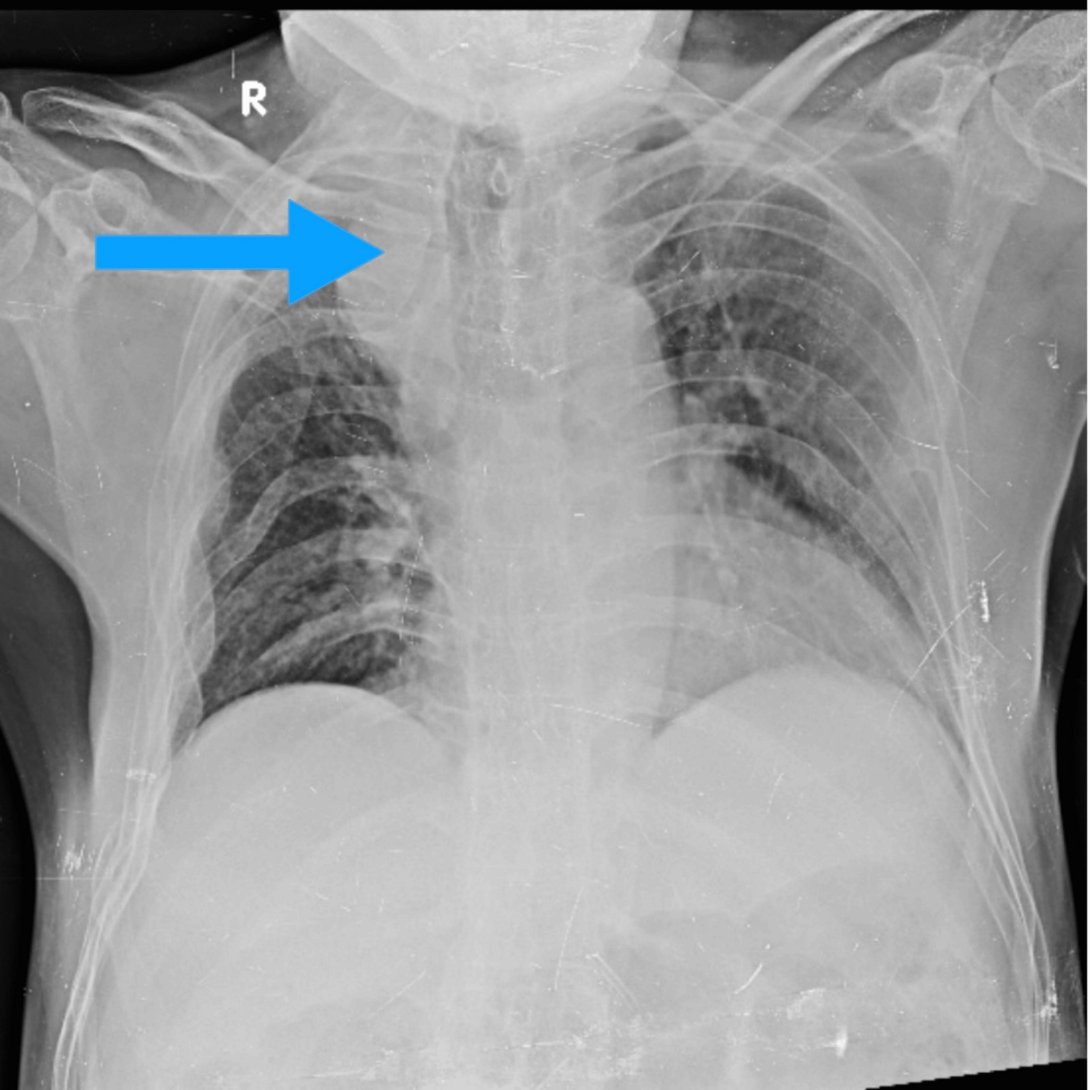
AP chest radiograph showing increased upper thoracic kyphosis. There is a probable right paratracheal opacity mass with tracheal deviation The blue arrow shows the opacity which might indicate the mass. AP: anteroposterior
